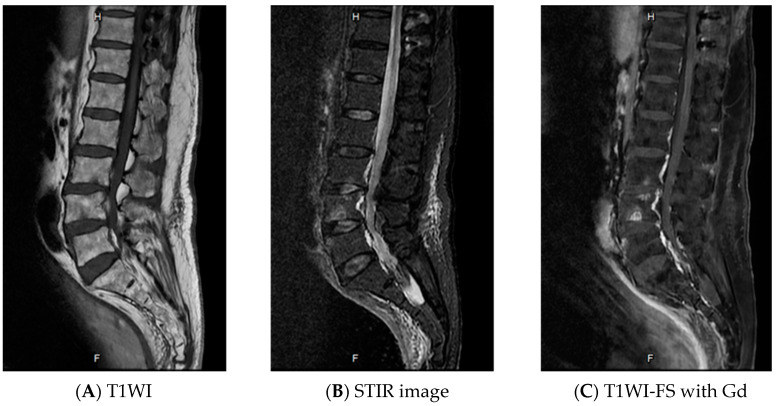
Magnetic resonance imaging of the lumbosacral spine at the 16th month of treatment showing complete resolution of the lesion.
